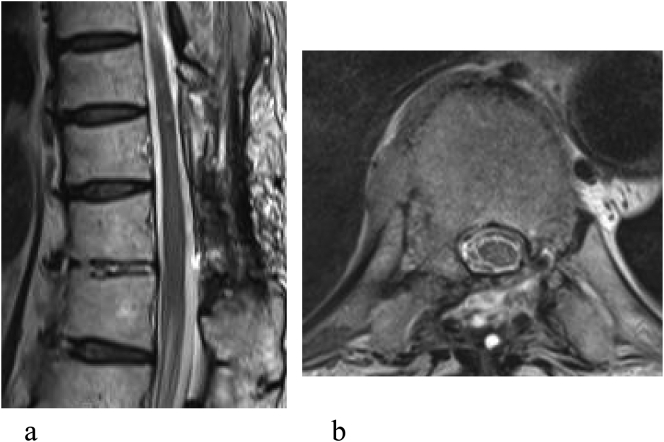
Postoperative T2-weighted magnetic resonance scan showing resolution of the pseudomeningocele. (a) Sagittal view. (b) Axial view.
